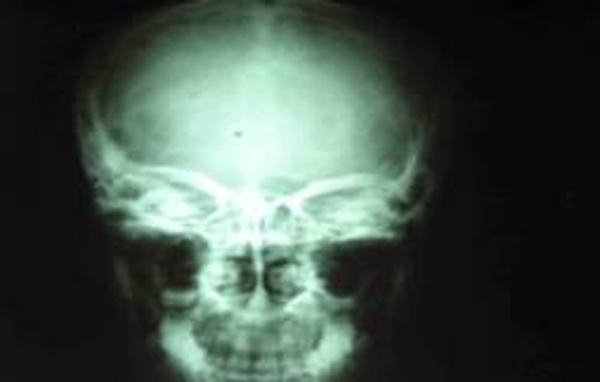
X-ray of the skull showing a well defined area of radiodense shadow overlying the right frontal bone with sella appearing normal.
